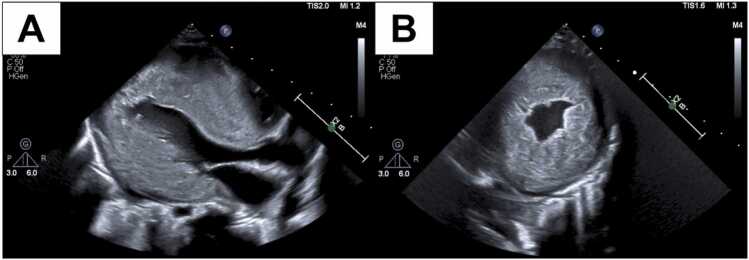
Initial transthoracic echocardiogram. (A) Parasternal long and (B) short axis views demonstrating significant concentric LVH without LVOT obstruction based on spectral Doppler mapping (not shown).
